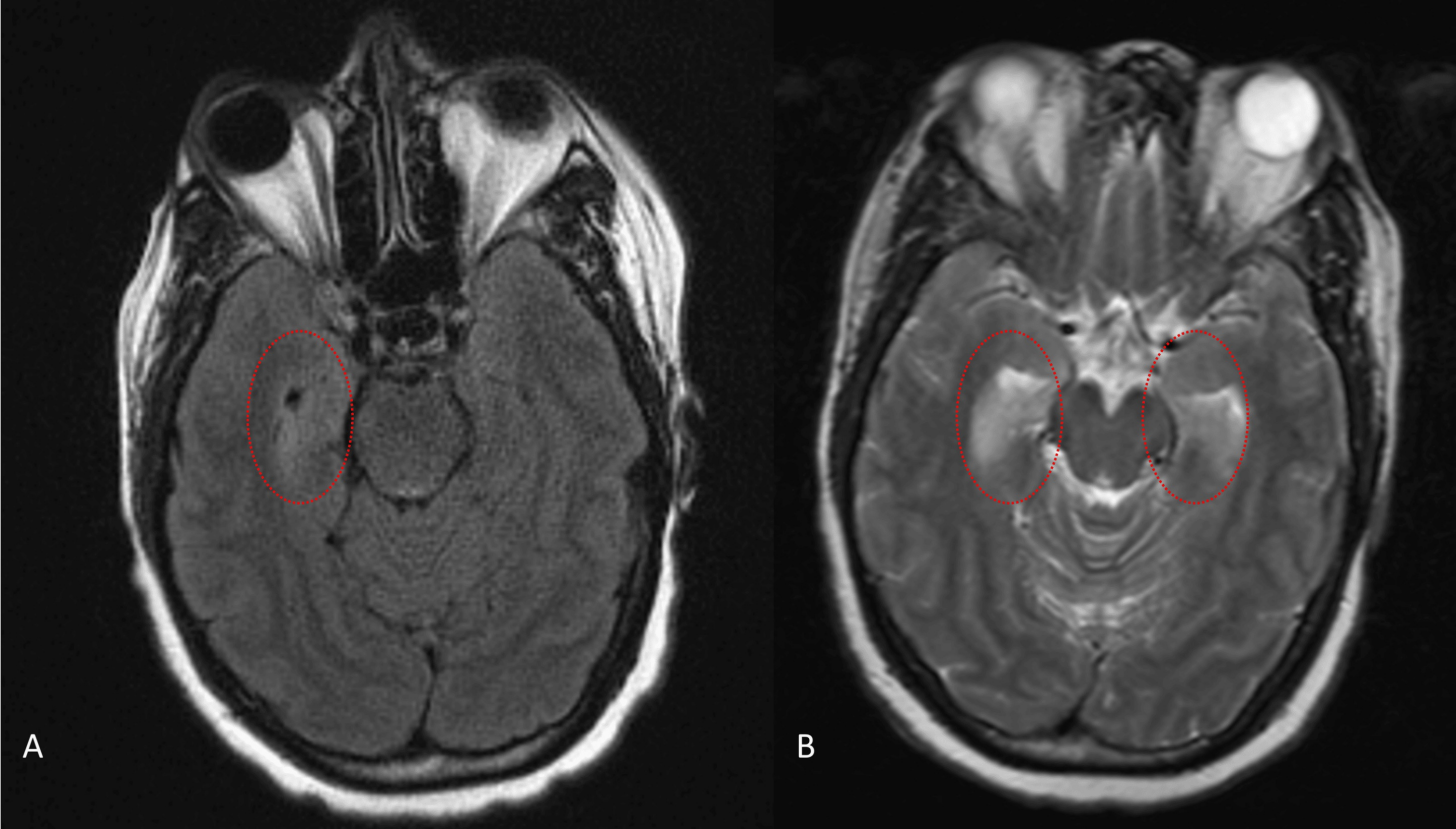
Case 2 brain MRI with signs of AE (A) Case 2 initial brain MRI axial view of the T2 FLAIR sequence revealing hyperintensity involving the right mesial temporal lobe. (B) Case 2 subsequent brain MRI axial view of the T2 FLAIR sequence revealing worsening hyperintensity involving bilateral mesial temporal lobes, suggestive of limbic encephalitis AE, autoimmune encephalitis; FLAIR, fluid-attenuated inversion recovery
